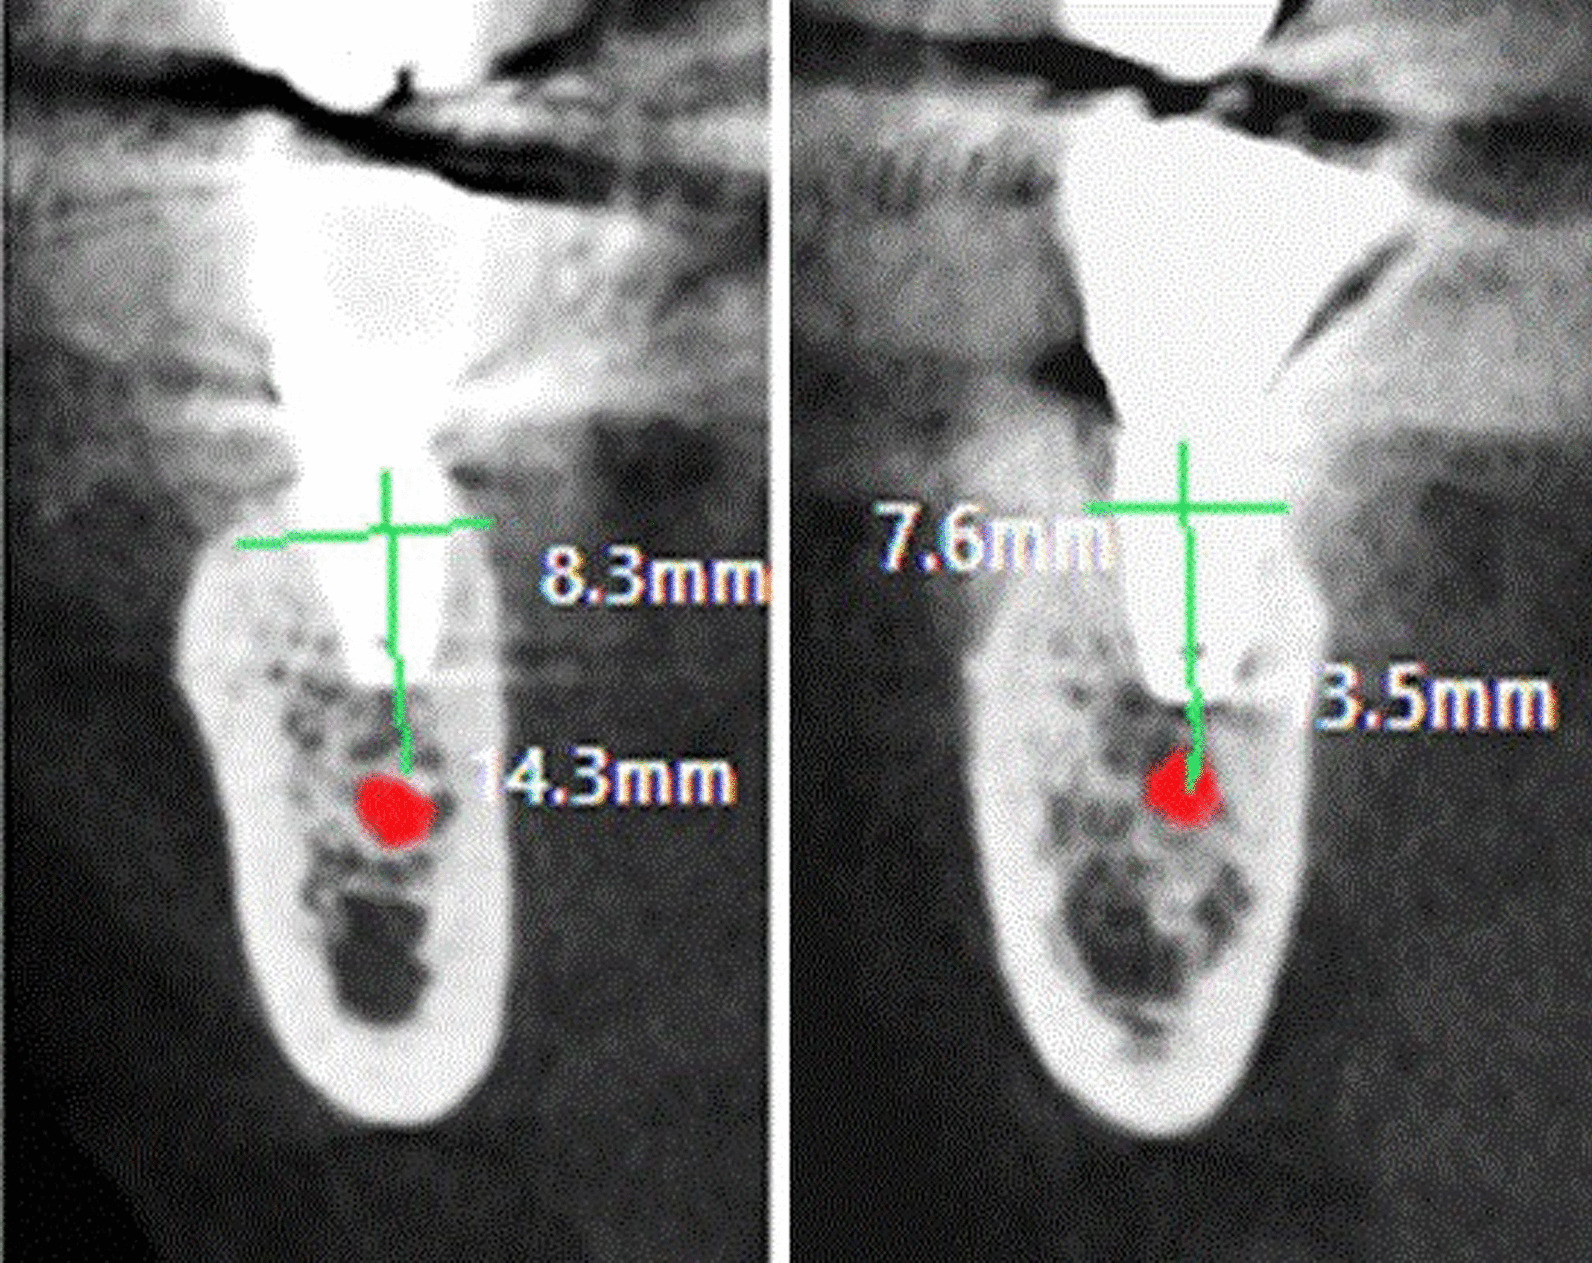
CBCT shows implant after 6 months from its placement
